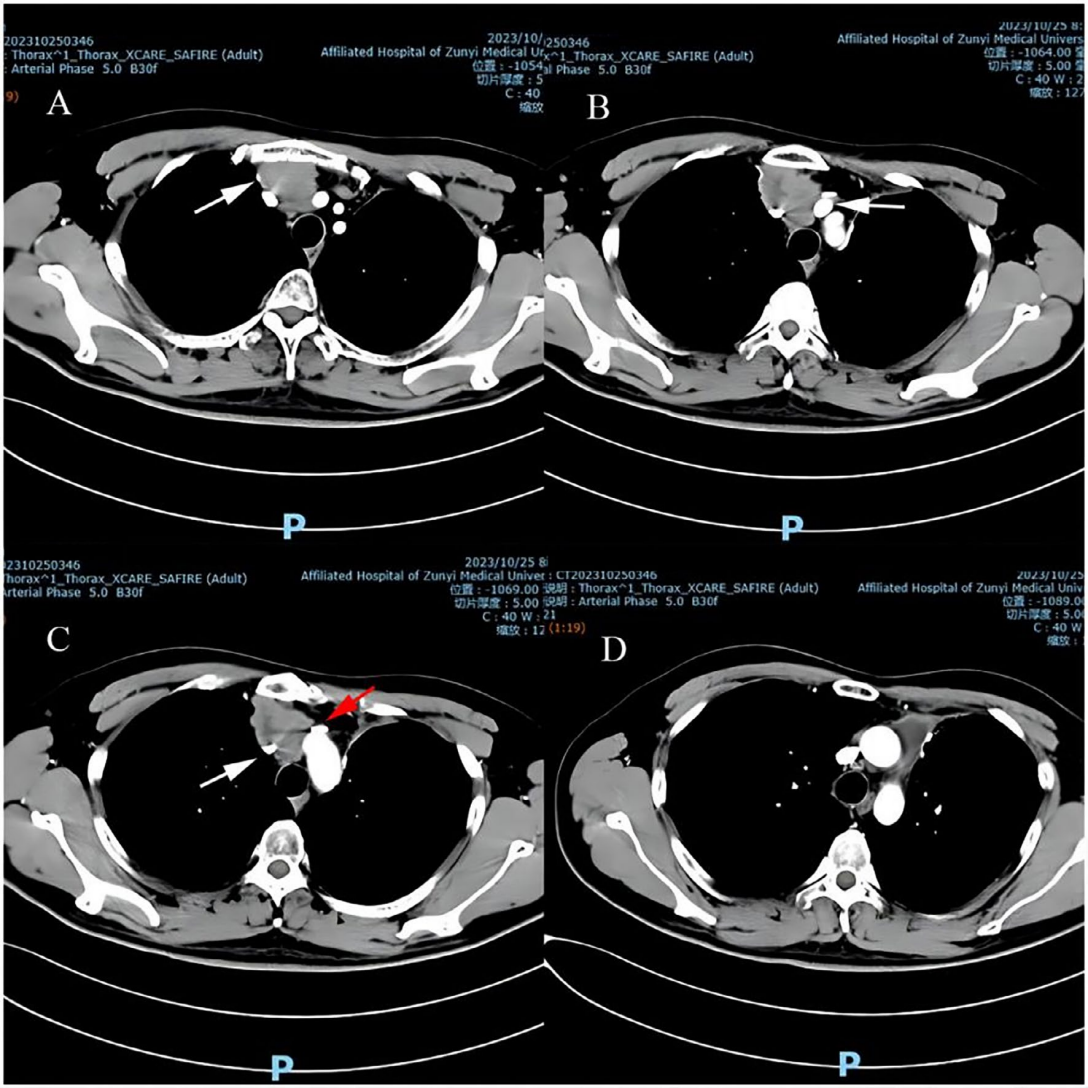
Time‐lapse computer tomography (CT) imaging of the chest of the patient with thymoma. (A) White arrow indicates right brachiocephalic vein. (B) White arrow indicates brachiocephalic trunk. (C) White arrow indicates superior vena cava, red arrow indicates left brachiocephalic vein. (D) Continuation of the C layer and can be left unmarked and uninterpreted.
